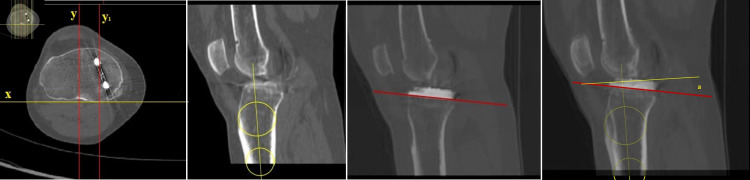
Sagittal alignment on CT scans At the level of the line connecting the posterior tubercles of the tibia, another line was drawn perpendicular to that. The sagittal slices were obtained in reference to this perpendicular line. The midsagittal slice that crosses through the intercondylar eminentia where the tibia is at its widest and the sagittal slice that crosses through the implant were overlapped. Then, sagittal measurements were performed with the circle method described in the text CT: computed tomography
